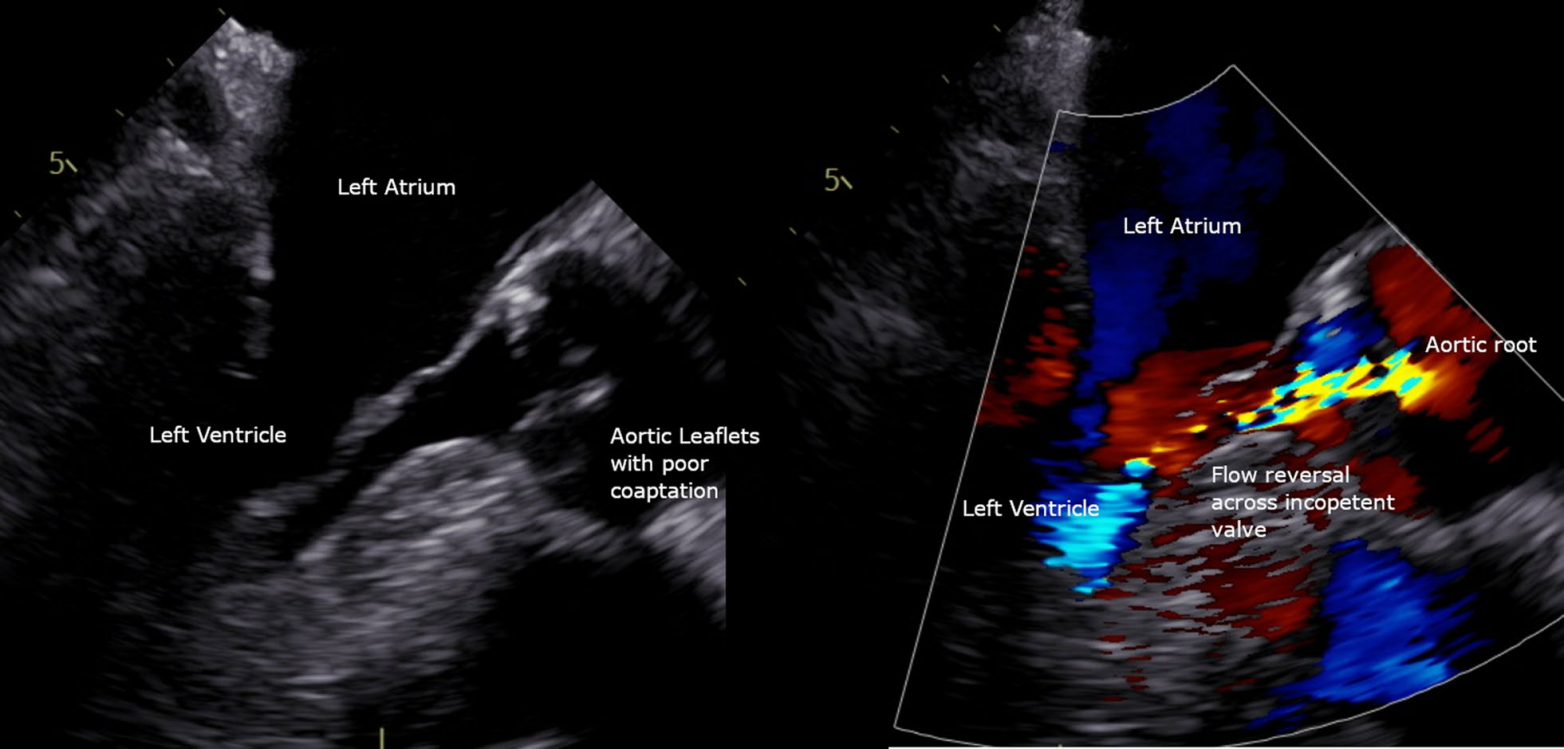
Preoperative Transesophageal Echocardiogram demonstrating insufficient aortic valve leaflets, with incomplete coaptation during diastole (left) and color doppler demonstrating flow reversal across the valve during diastole (right)
